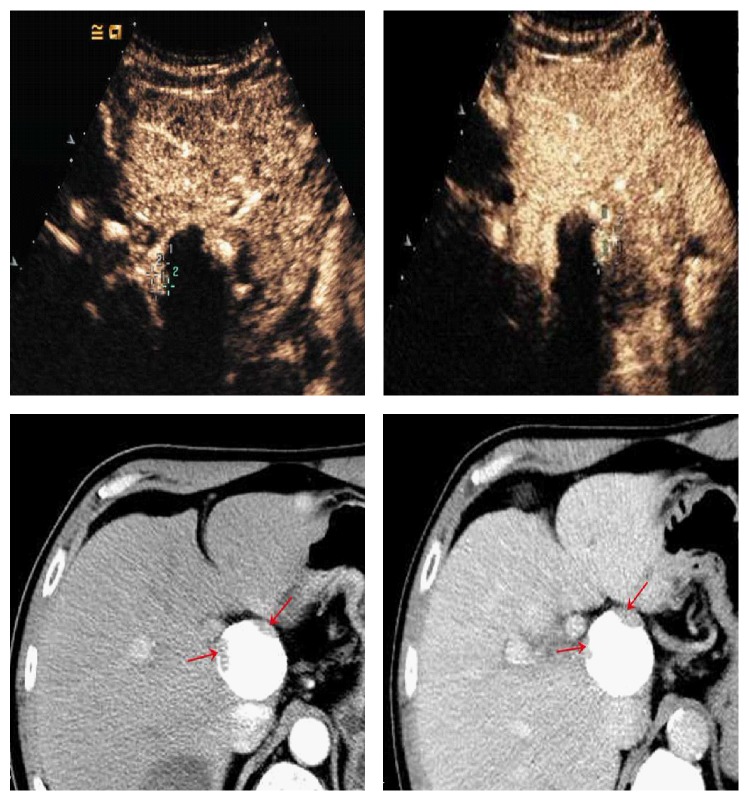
53-year-old man with 4.8cm×4.0 cm tumor. Nodular enhancement (cross mark) seen on CEUS (a, b) was consistent with the contrast-enhanced CT images (c, d) (red arrows).
